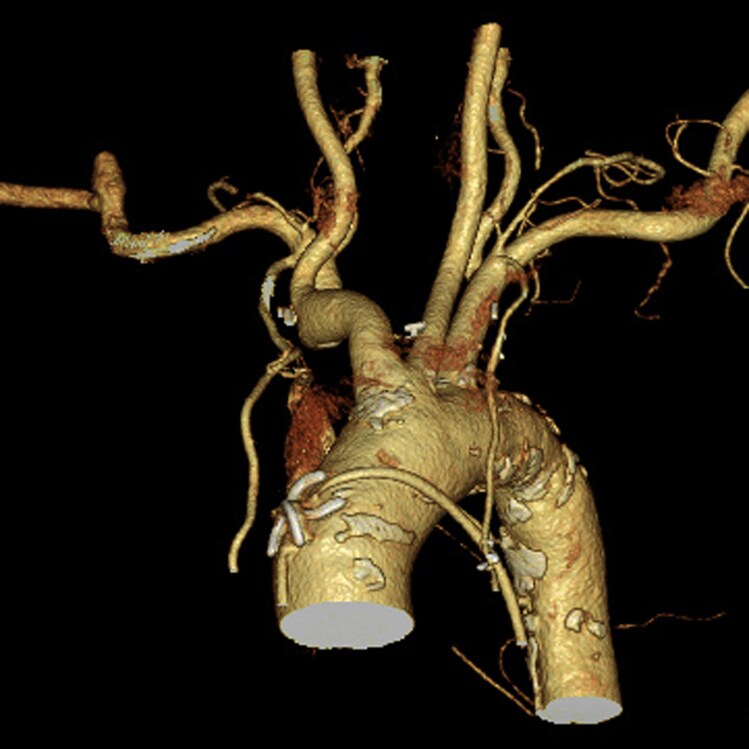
Post-operative computed tomography confirmed the absence of vascular injury.
